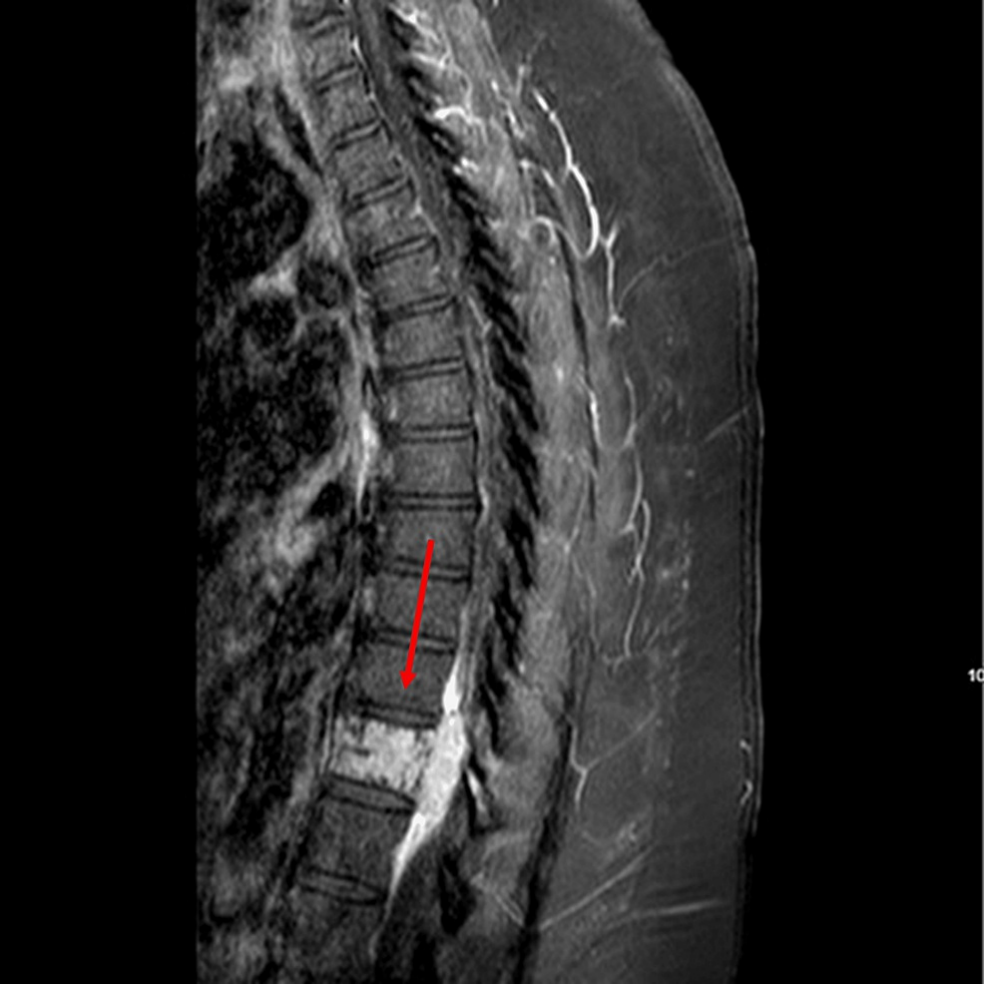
MRI thoracic spine with and without contrast Marked abnormality of the T11 vertebral body with diffuse enhancement and significant epidural disease in the spinal canal. Epidural soft tissue related to the T11 lesion (arrow) results in severe central canal stenosis and cord compression.
